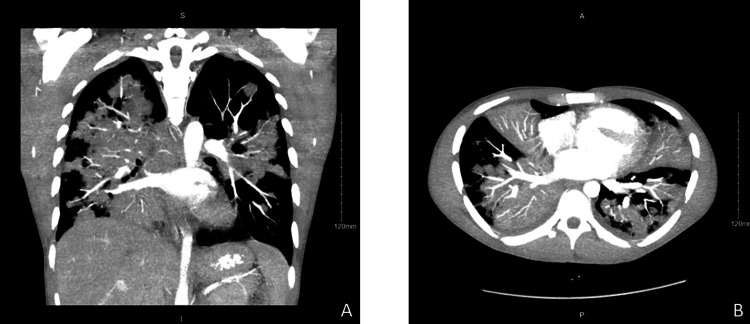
Computed tomography scan pulmonary embolism protocol Significant consolidation is noted in the bilateral lungs (right greater than left), which appears slightly worsened compared to the prior examination. A: coronal view; B: axial view.
